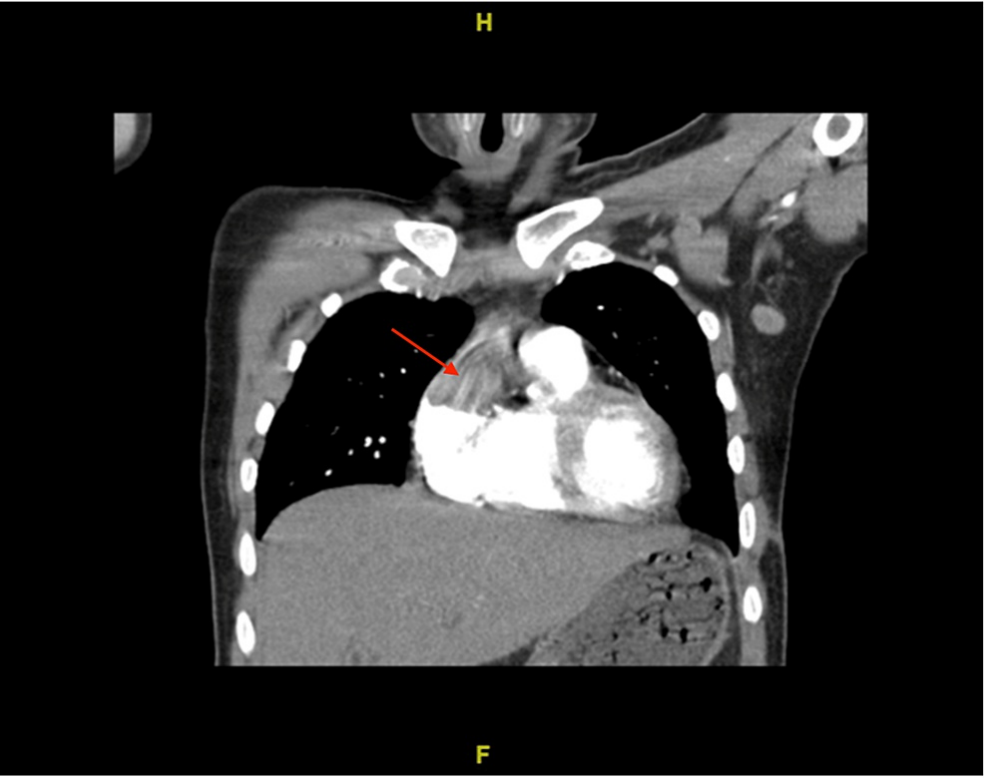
Chest CT with angiography revealing intracardiac mass in the right atrium (red arrow)
